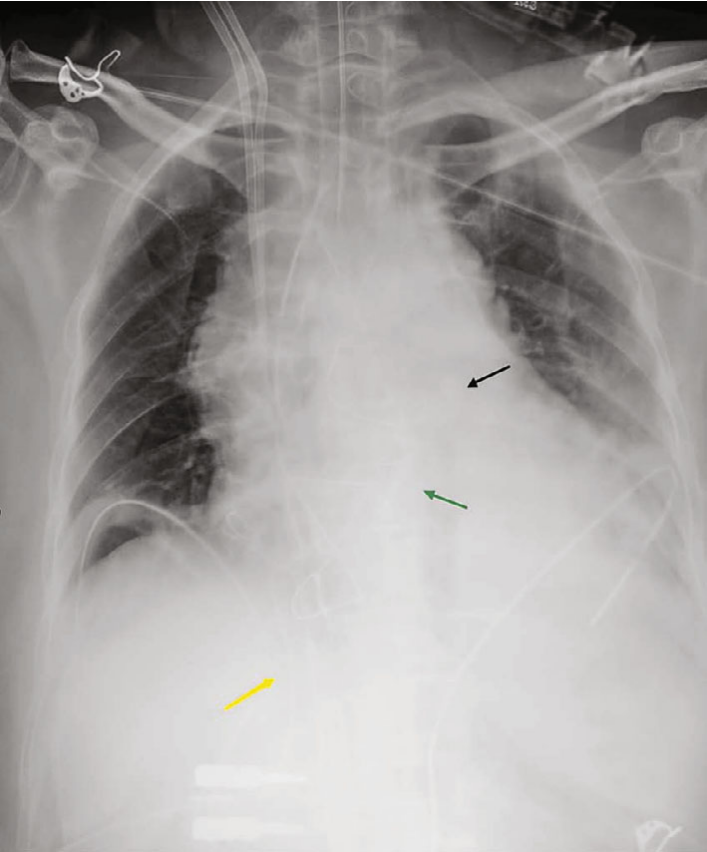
Chest X-ray upon arrival. Black arrow: intra-aortic balloon pump (IABP); yellow arrow: drainage venous cannula; and green arrow: bioprosthetic valve.
